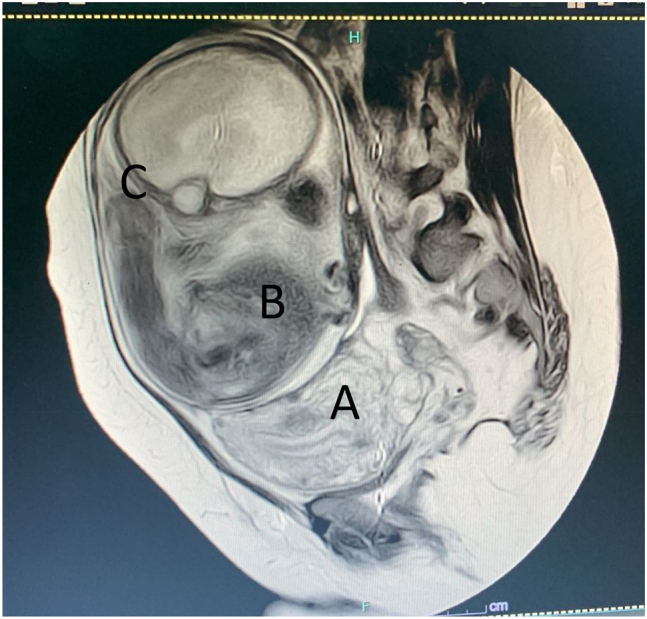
Magnetic resonance imaging showing (A) intact uterus, (B) placenta, (C) extrauterine fetal sac with viable fetus.
